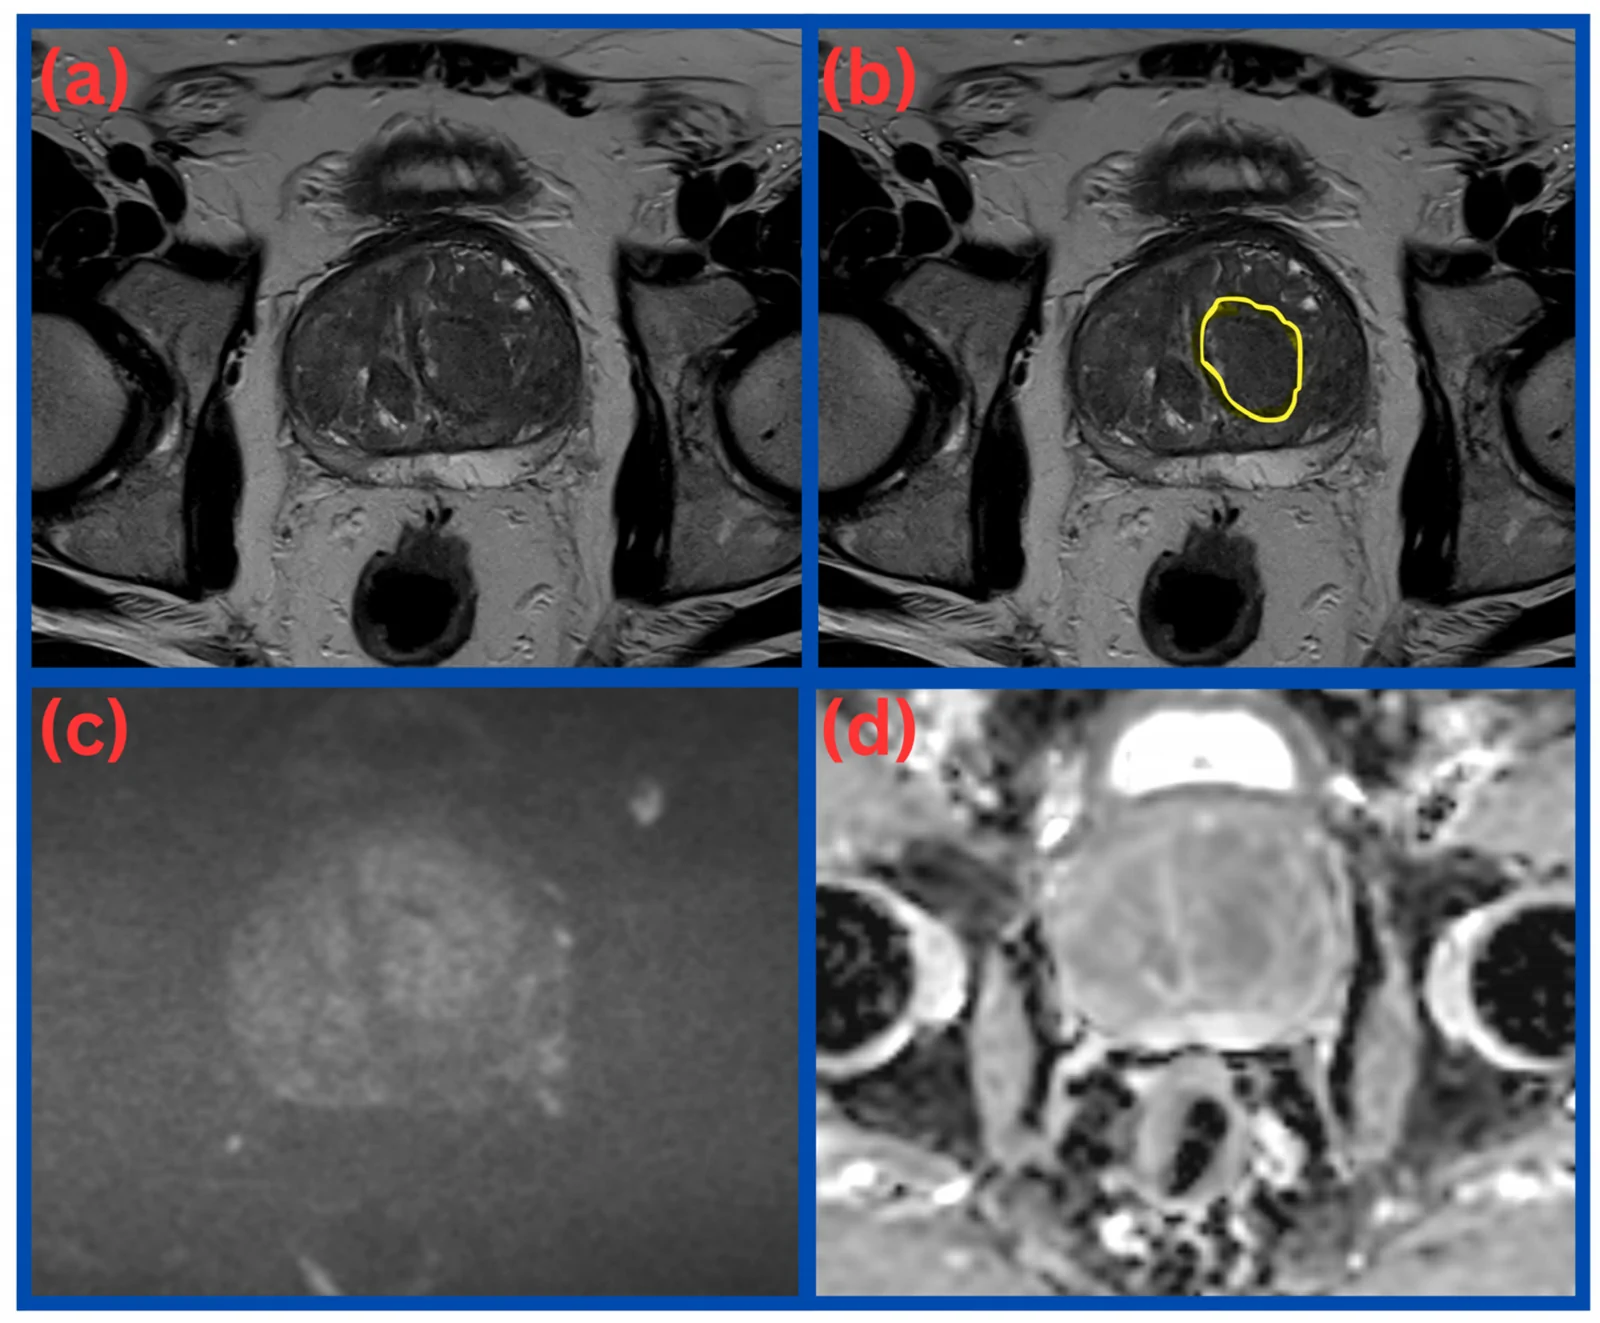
Multiparametric MRI of a PI-RADS 2 appearance in the transition zone. (a) Axial T2-weighted image showing multiple well-circumscribed cystic and nodular changes with only mild T2 hypointensity, measuring up to approximately 25 mm and typical of benign nodular hyperplasia, and (b) the corresponding axial T2-weighted image with the region of interest outlined in yellow. (c) High *b*-value diffusion-weighted image (*b* = 1400 s/mm^2^) and (d) the apparent diffusion coefficient (ADC) map demonstrates no convincing diffusion restriction corresponding to the T2 changes. The combination of well-defined margins, encapsulated morphology, and absence of a diffusion correlate is characteristic of a PI-RADS 2 (clinically significant cancer unlikely) assessment.
